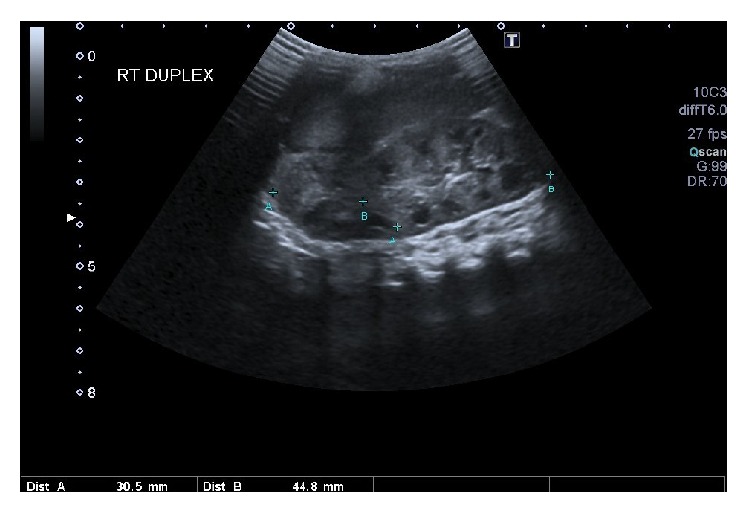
Ultrasonography of the right kidney at 12 days of age showing evidence of duplex system. The kidney was visualised within the right renal fossa.
